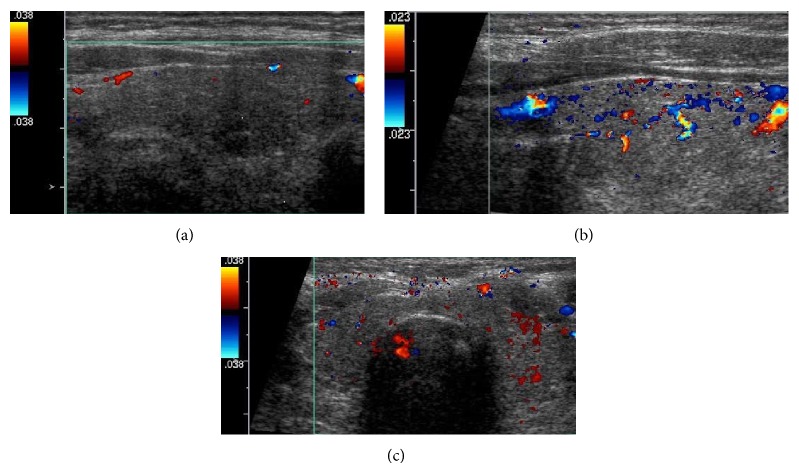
Thyroid ultrasound with color Doppler flow study showing normal to minimally reduced vascularity of the glandular tissue. (a) Long axis view of the right thyroid lobe. (b) Long axis view of the left thyroid lobe. (c) Transverse axis view of both lobes of thyroid gland including the isthmus.
